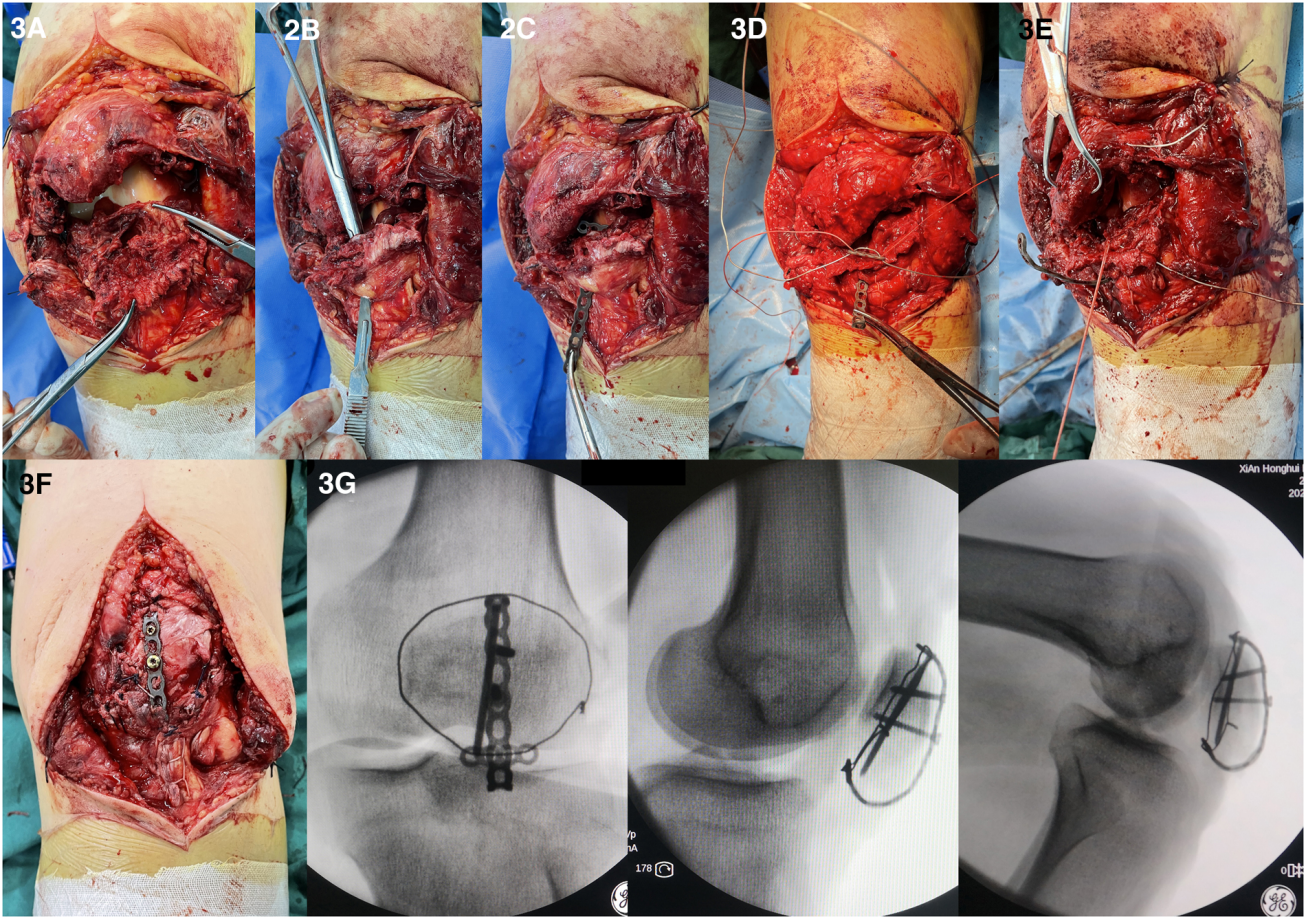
(A) The patellar tendon is broken from the lower pole of the patella, and the lower pole is avulsion fracture; (B) make a small opening in the distal patellar tendon; (C) the T-shaped plate reversely passes through the orifice, and the transverse arm is hung on the patellar tendon; (D) the steel wire and suture pass through the transverse arm hole of the plate for use; (E) the suture “Z” weaves the distal patellar tendon and passes through the transverse arm hole of the plate for use; (F) fix the plate with screws after tightening the steel wire and knotting the suture; (G) the x-ray fluoroscopy showed good fixation and internal fixation.
